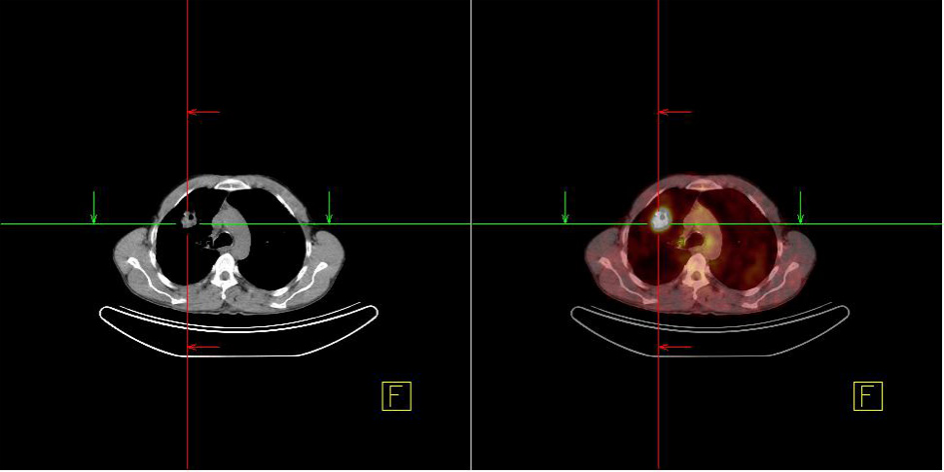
Lung mass detected in the PET/CT imaging.
